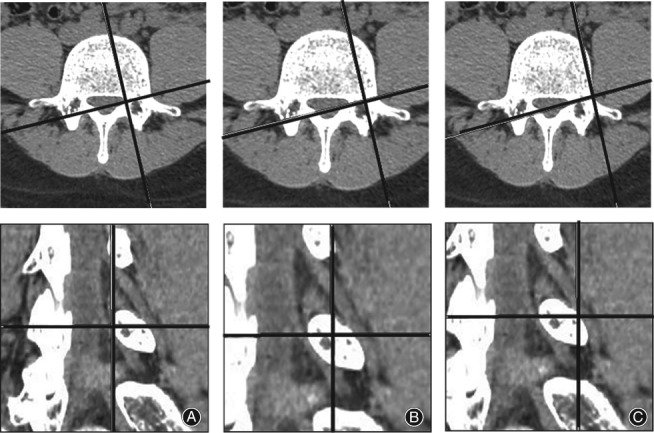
Examples of the medial edge of the pedicle (A), the middle of the pedicle (B), and the lateral edge of the pedicle (C), as located on axial and coronal CT images.
